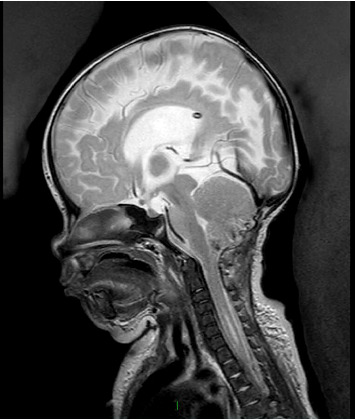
Three-month postoperative T2 weighted sagittal MRI demonstrating resection of the tumour and improvement in cervical syringomyelia (from patient notes).
